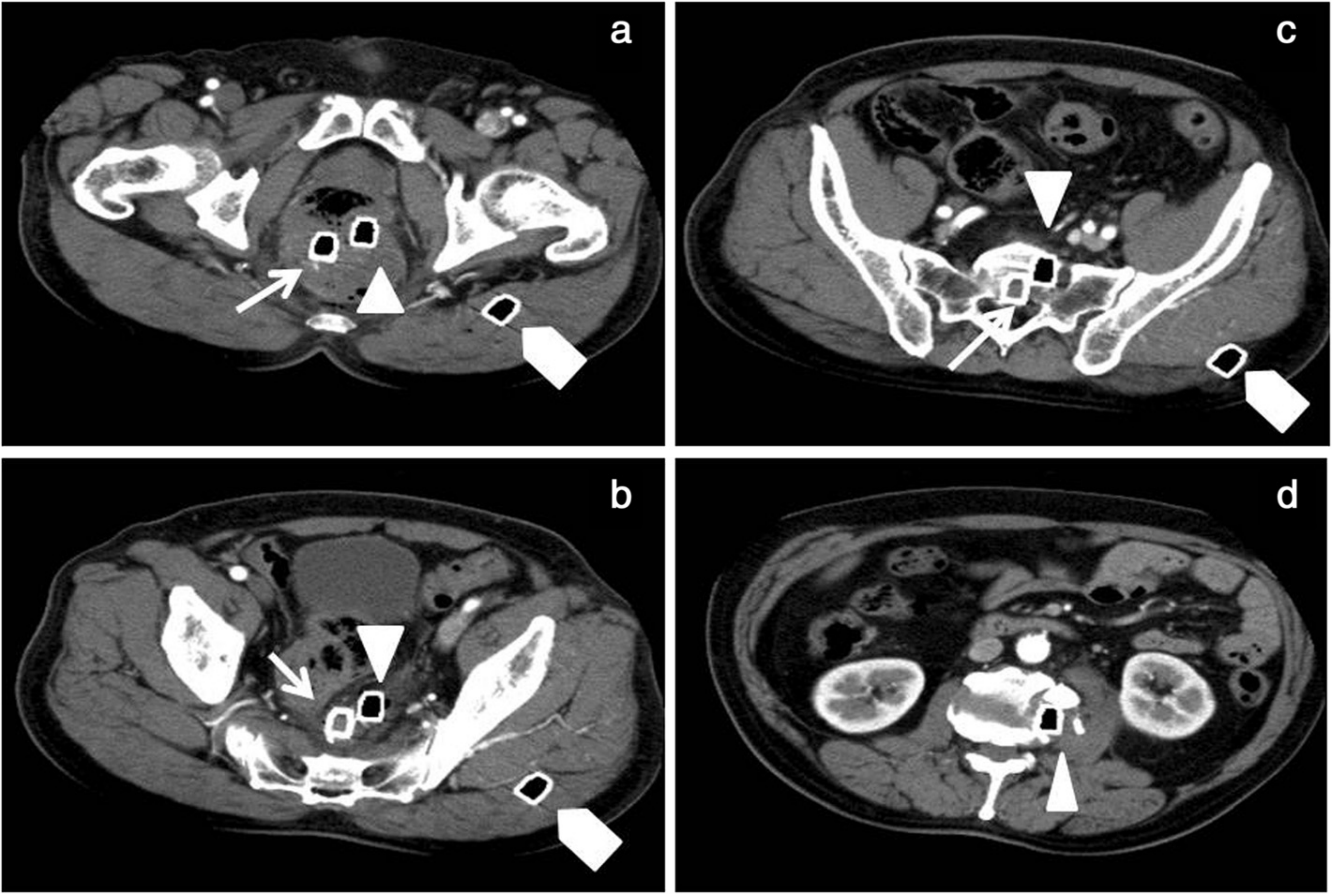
a-d. Abdominal computed tomography images (axial view) with three steel bars: bar A (⌂), bar B (∆) and bar C (↑).
